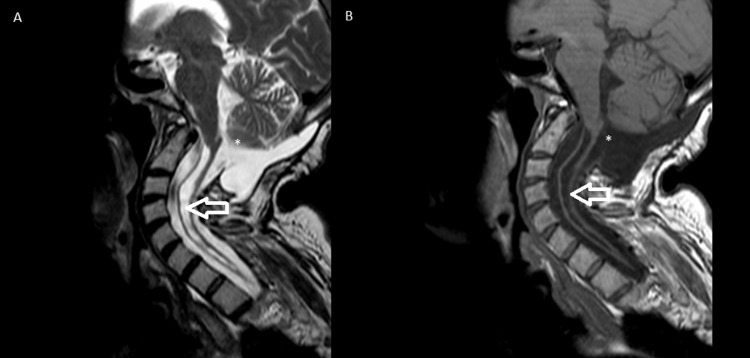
Magnetic resonance imaging, T2-weighted (A) and T1-weighted (B), performed 1 year after surgery Postoperative changes following foramen magnum decompression (white asterisk) and reduction of the syringomyelic cavity previously observed in the preoperative scan (white arrow)
